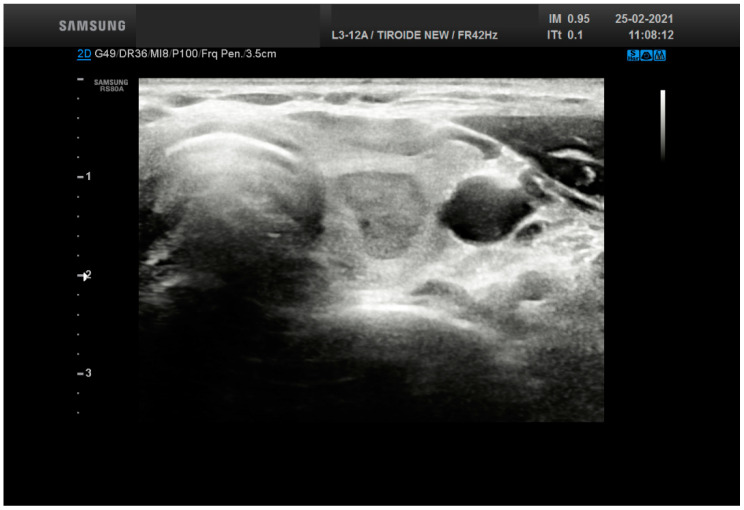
Ultrasound features from a thyroid nodule (15 mm size) resulting into a score 7 belonging to TR 5 (solid, very hypoechoic, lobulated). The lesion was diagnosed as Follicular nodule.
